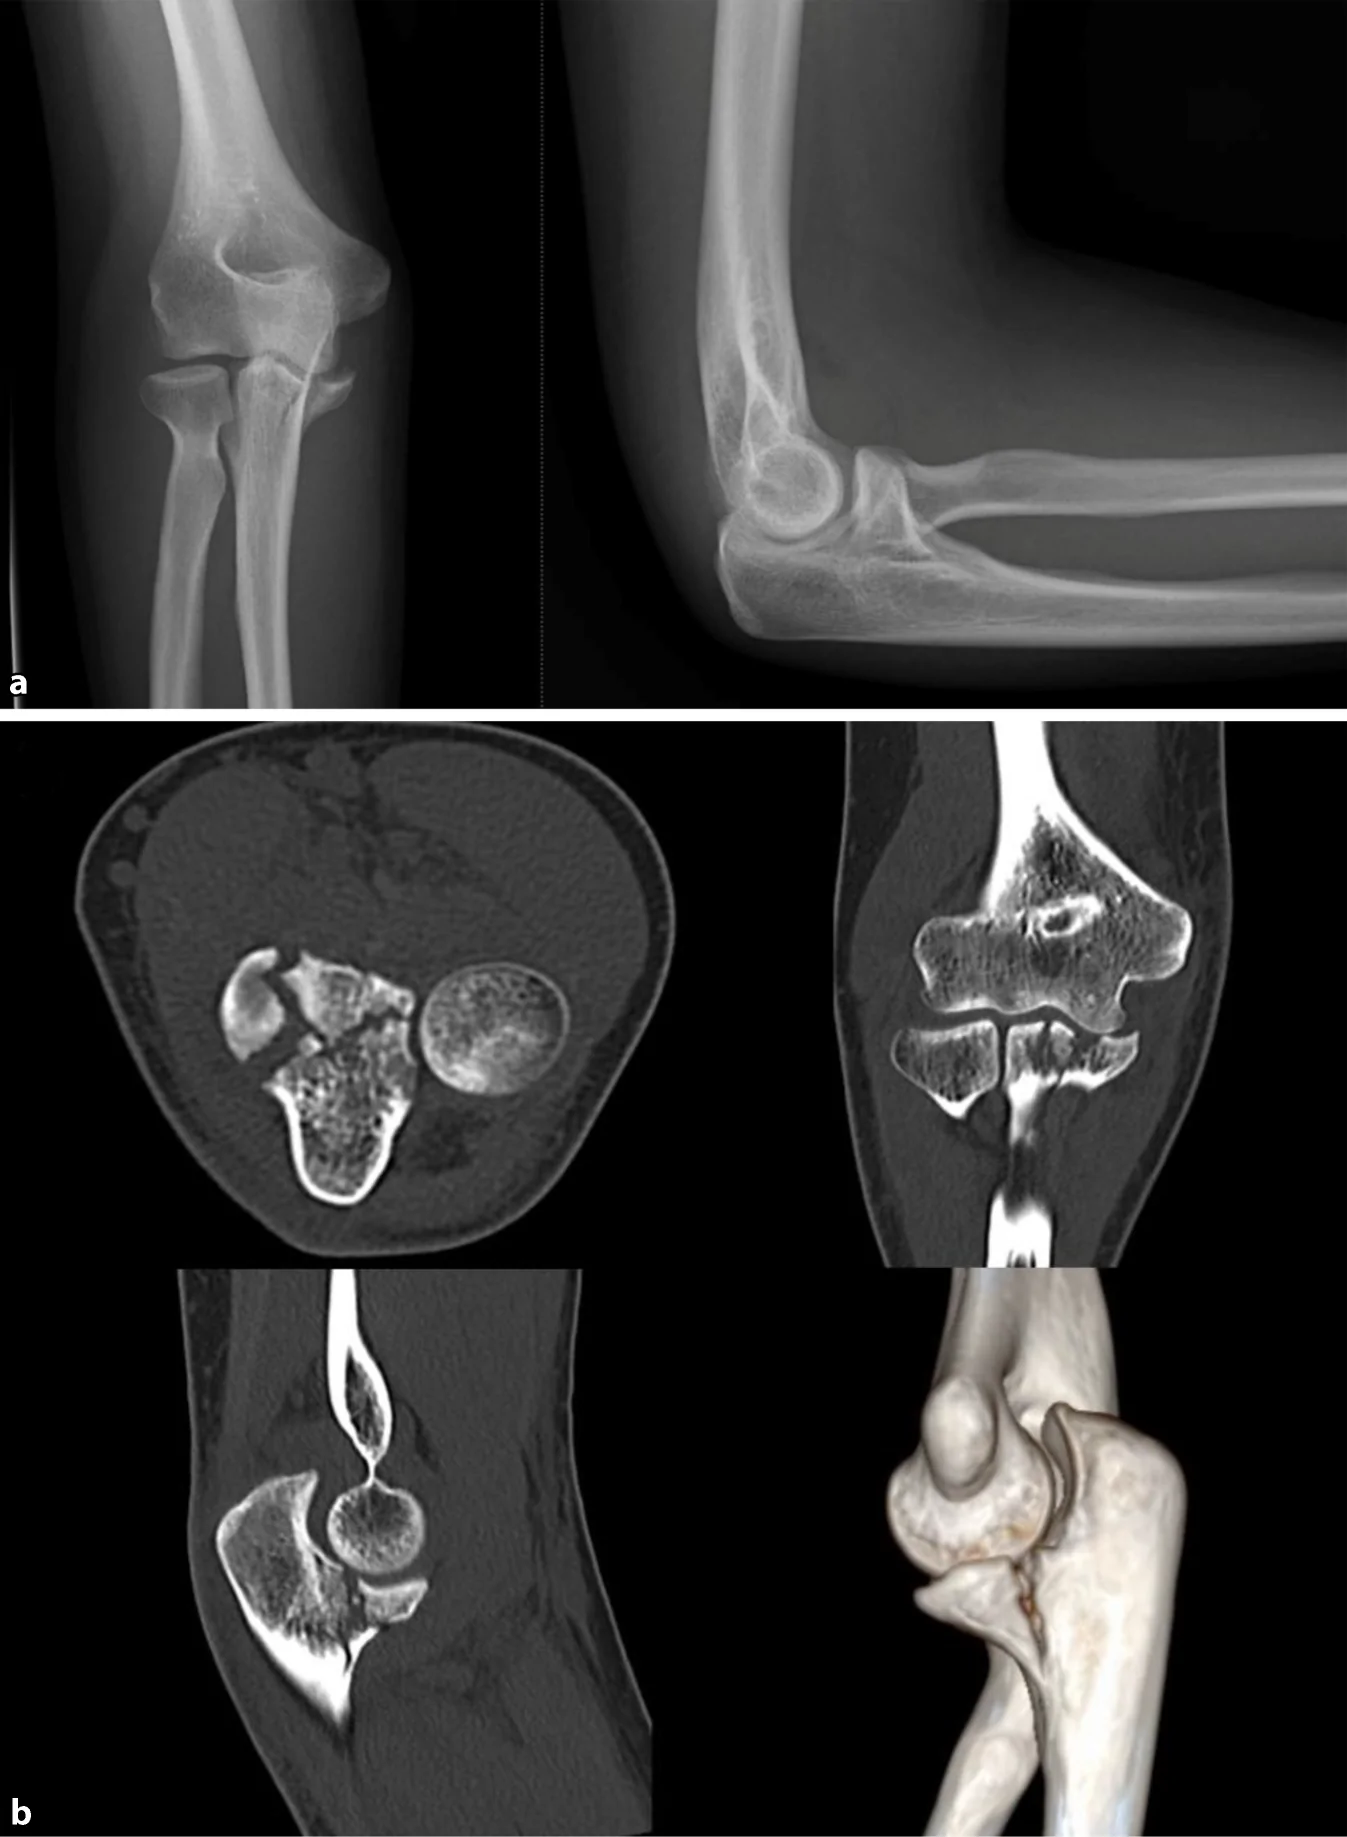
Radiographs (a) and computed tomography (b) demonstrate an O’Driscoll basal type 1 fracture of the coronoid body and base with extension into the lesser sigmoid notch. After fracture debridement and provisional fixation, a lag screw and buttress plate were selected to fix the multifragmentary coronoid base fracture (Fig. 11)
